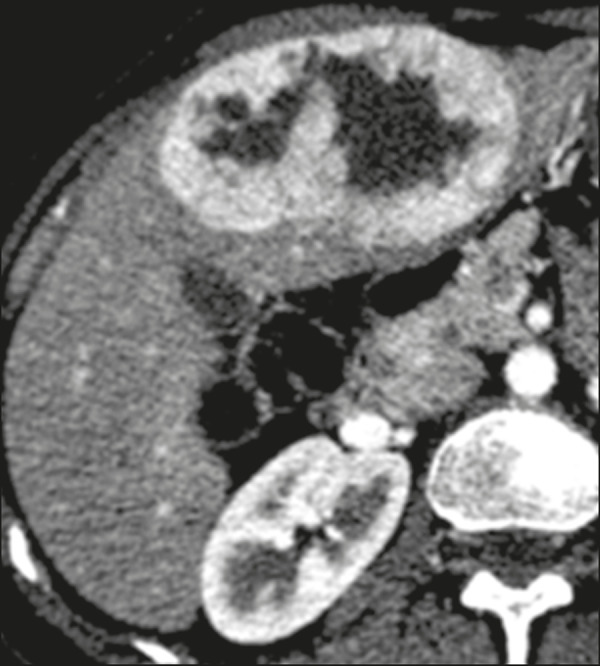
PHNEN. An arterial phase CT scan showing a large heterogeneous liver mass with a hypervascular enhancement pattern and a necrotic component.
